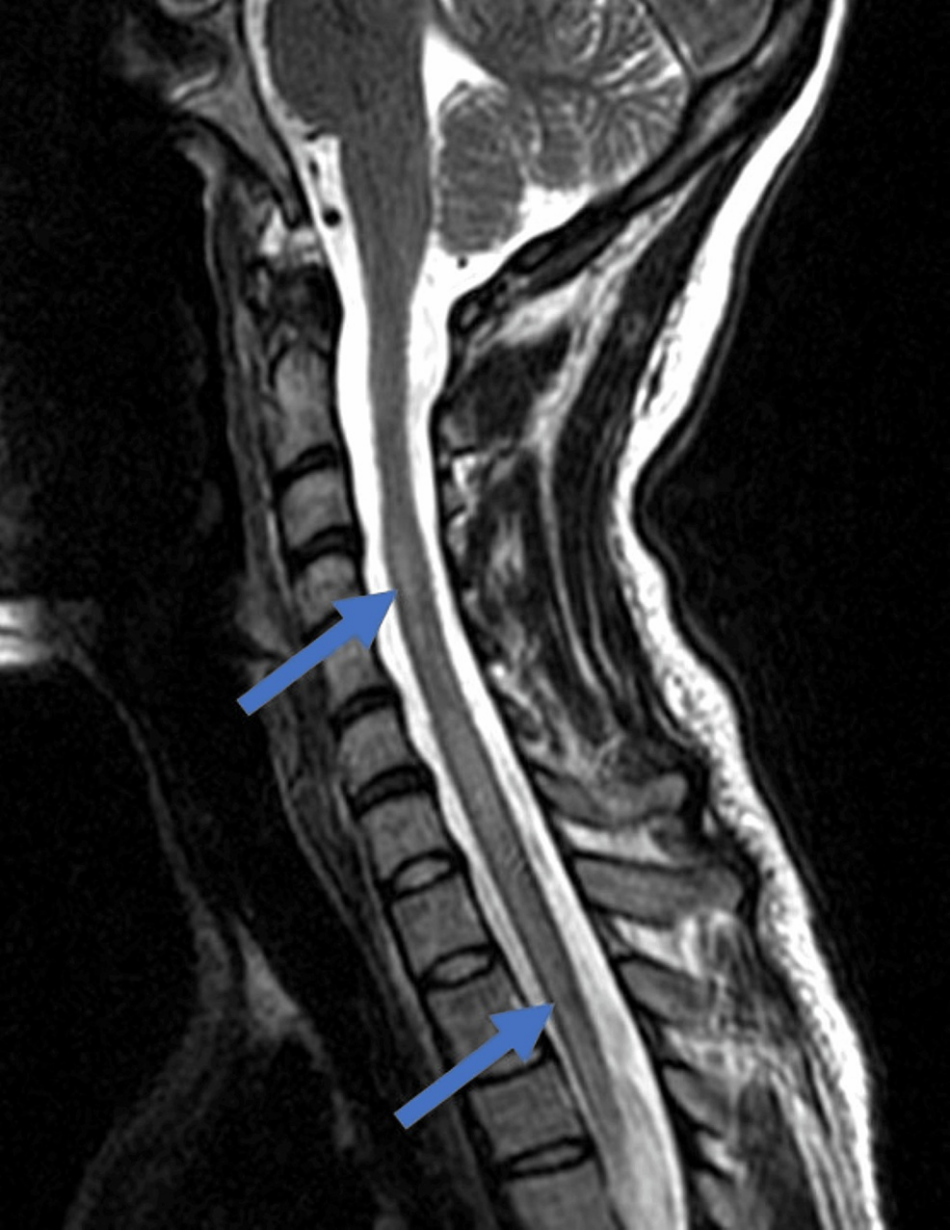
T2-weighted spine MRI demonstrating hyperintense signals in the cervical and thoracic spine (blue arrows). MRI: magnetic resonance imaging
